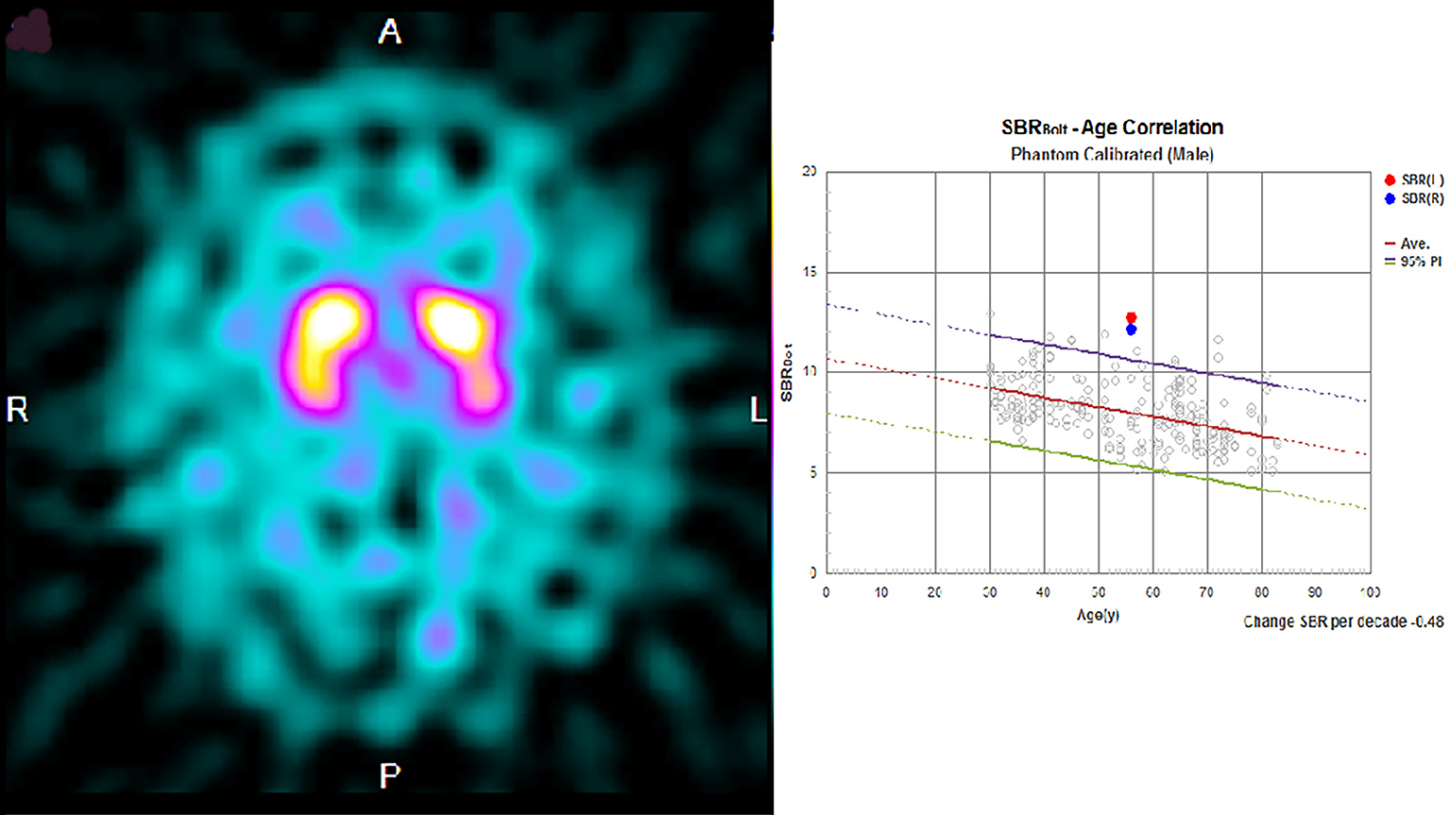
Dopamine transporter single-photon emission computed tomography imaging using ioflupane I 123 injections. Normal finding of the patient diagnosed with diabetic uremic syndrome.
